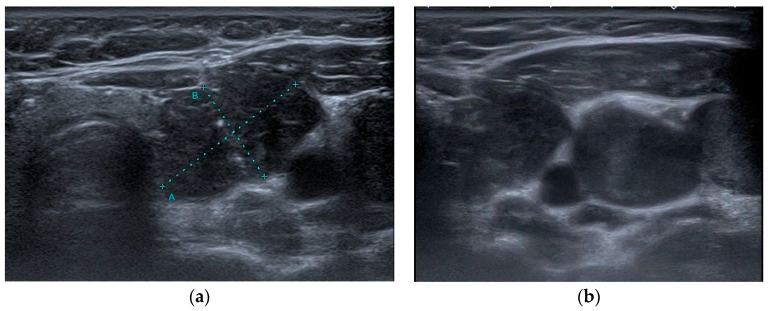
On the left (a), a gray-scale transverse ultrasonographic image shows an irregularly contoured lesion in the left thyroid lobe, measuring 26 × 17 mm in its largest dimension (A: length, B: width), extending beyond the thyroid capsule into the left anterolateral neck, obliterating the lateral carotid space and anterior scalene muscle planes. FNAB classified the lesion as Bethesda Category VI, with postoperative pathology confirming papillary thyroid carcinoma. On the right (b), a markedly hypoechoic nodule with irregular margins is observed in the left thyroid lobe, accompanied by two adjacent nodular metastatic lymph nodes in the left cervical chain with obliterated fatty hilum, classified as suspicious. Postoperative pathology confirmed papillary carcinoma invasion in both lymph nodes.
